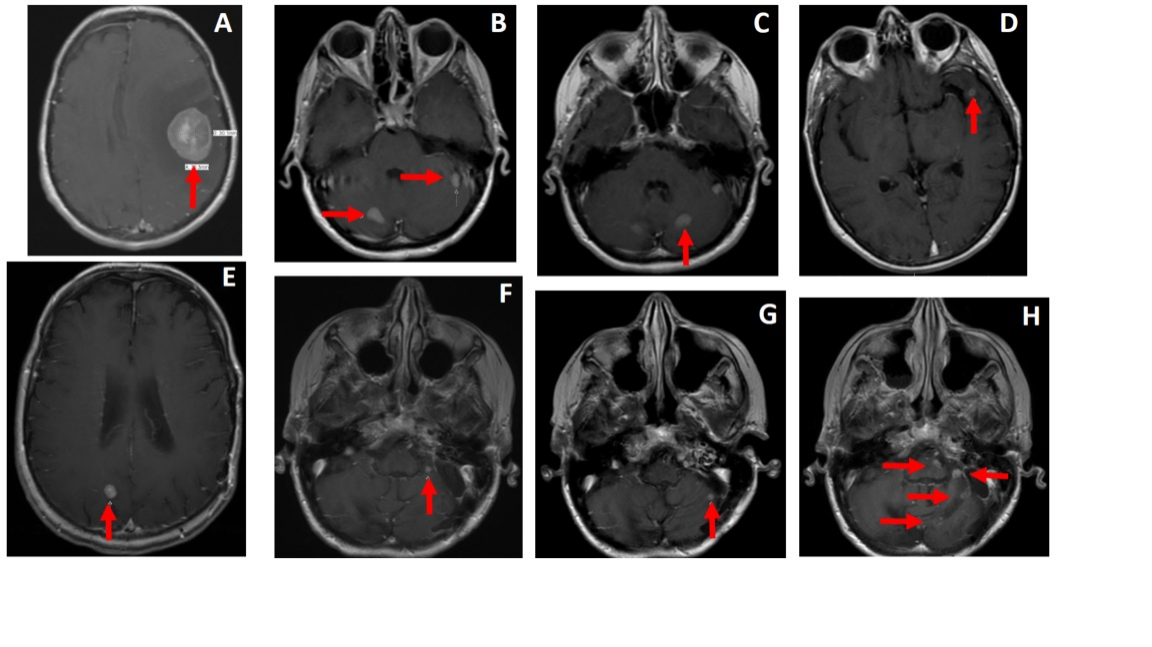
Pre-SRS treatment T1-weighted post-contrast brain MRI demonstrating intracranial metastasis Brain metastasis presenting time points include initial presentation 06/2006 (A&B), 03/2008 (C), 07/2009 (D), 02/2013 (E), 12/2013 (F), 11/2014 (G). Window (H) represents multifocal progression prior to the initiation of WBRT in 03/2015. MRI: magnetic resonance imaging; SRS: stereotactic radiosurgery; WBRT: whole brain radiation therapy.
